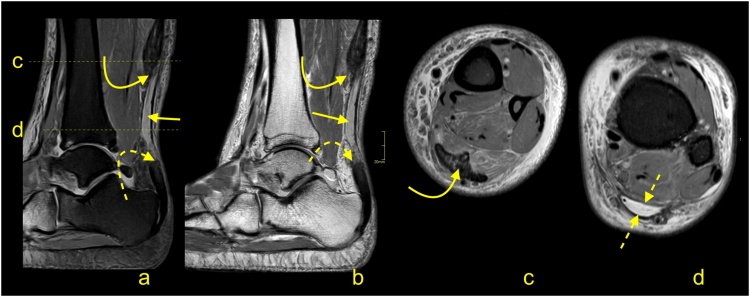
Chronic Achilles tendon rupture in midportion (straight arrow). A 46-year-old patient who suffered a ruptured Achilles tendon 2 years ago. MRI (a–d) revealed that the paratenon space is filled by fluid, and adhesions are visible between the proximal stump (curved arrow) and distal stump (dashed curved arrow). The paratenon is not distended, which indicates its chronic character (dashed arrow). A- T2- weighted with fat suppression, b- PD- weighted, c and d- PD- weighted with fat suppression.
